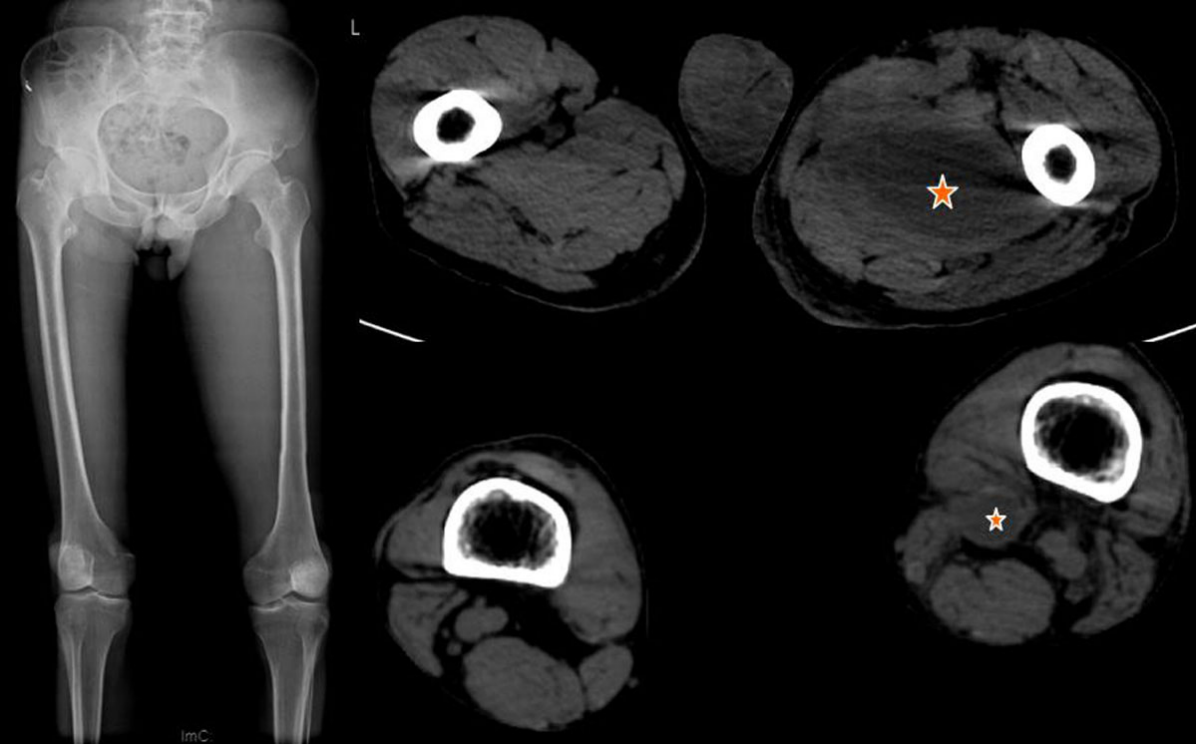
Plain film and CT of the lower limbs comparing the two sides. Plain film shows that medial aspect of the left thigh is swelling; CT shows the abscess involves left thigh adductors while the right thigh is normal.
